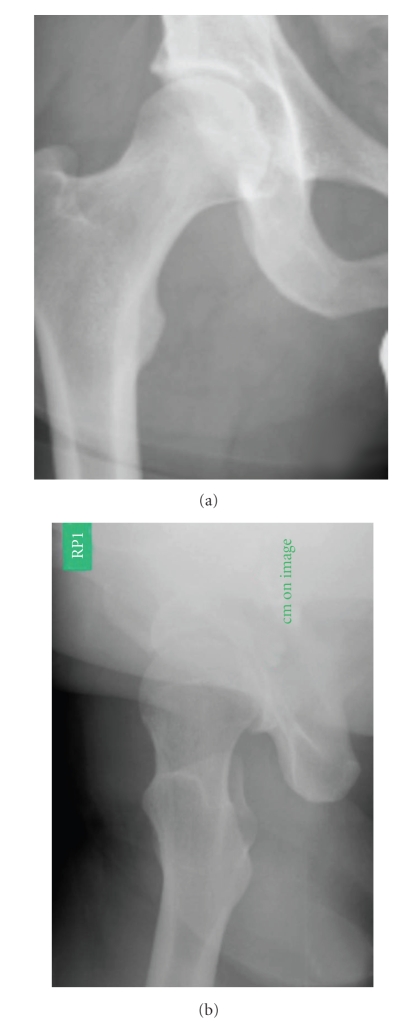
a/p view of the X-ray (a) and lateral view (b) of the right hip 6 months after closed reduction shows a congruent hip joint and no signs of AVN or osteoarthritis.
